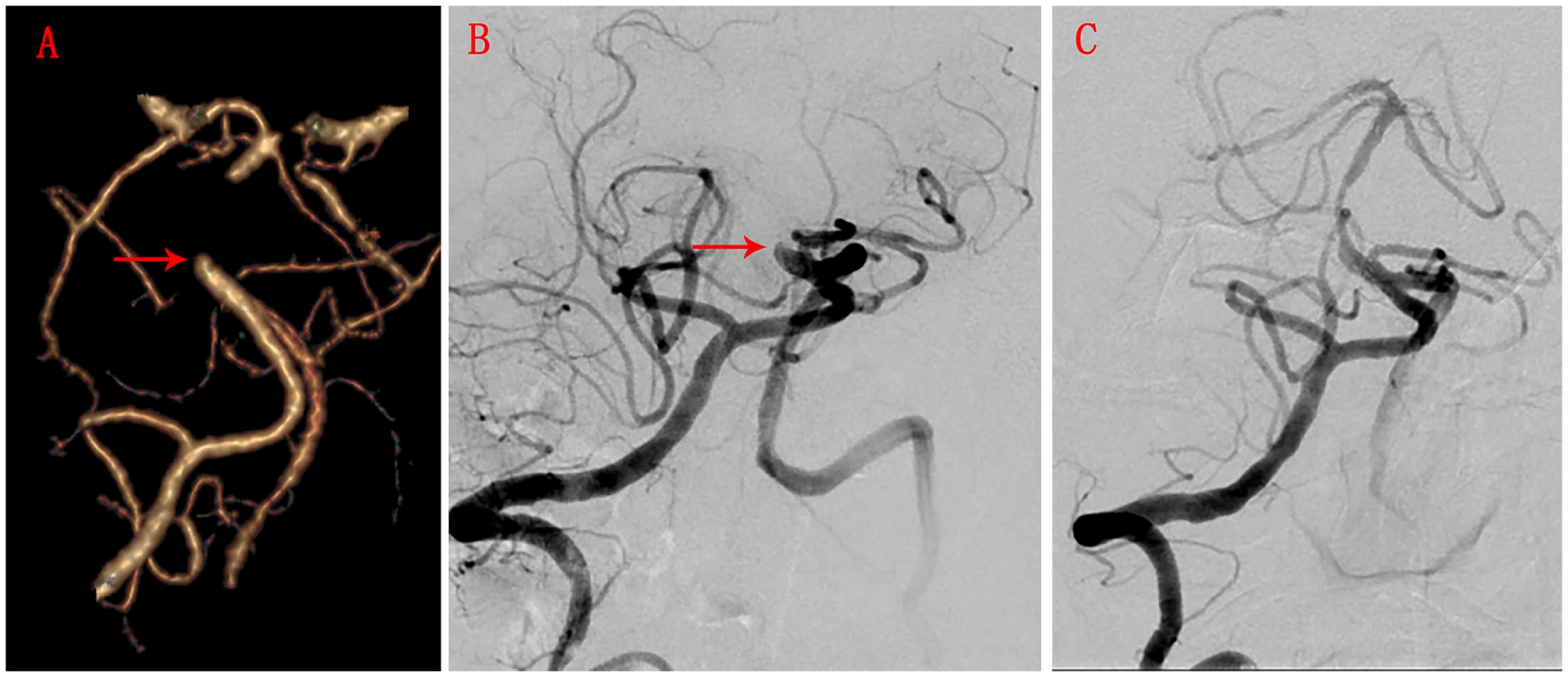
CTA and DSA images in case 1. (A) CTA shows the degree of basilar artery occlusion, the shape of the stump (red arrow), collateral circulation compensation, and the length of occlusion. (B) BSA shows the degree of basilar artery occlusion, the shape of the stump (red arrow), collateral circulation compensation, and the length of occlusion. (C) BSA after successful recanalization by endovascular interventional therapy.
